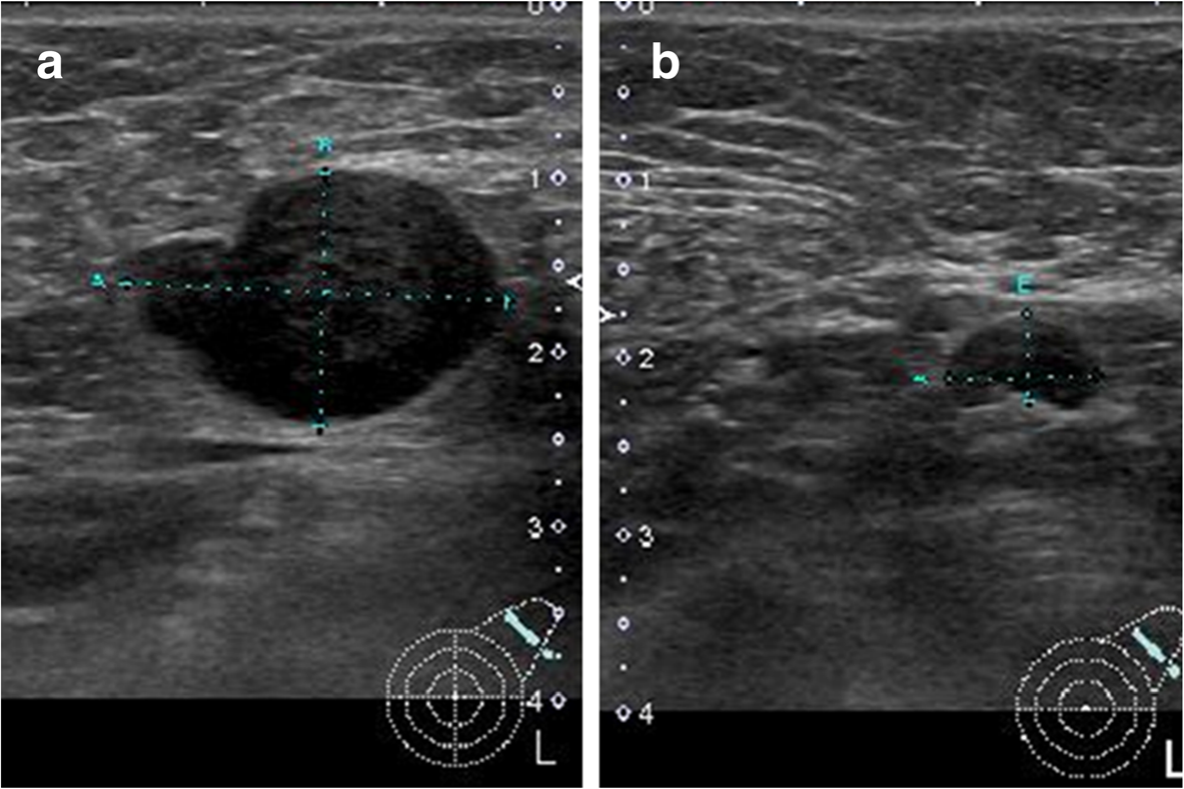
Ultrasound findings: Ultrasound of the *left axilla* showed multiple LNs with *irregular margins* in which central hyperechogenicity was lost (maximum size: 2.2 cm × 1.5 cm; a, b)
